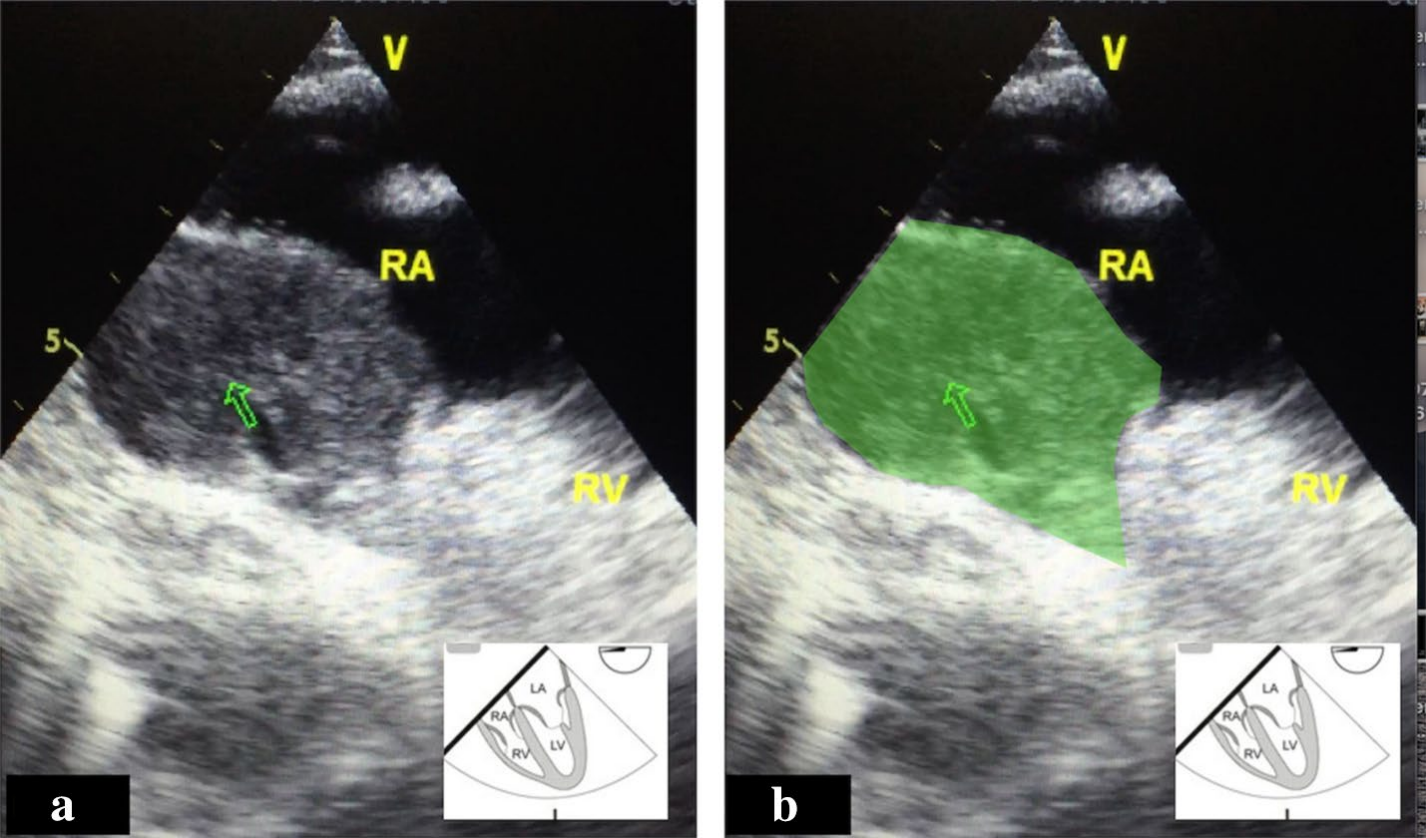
a, b Transesophageal echocardiogram (TEE) at mid-esophageal 4-chamber view with probe rotating to the right showed a compressed right atrium and a posterior pericardial clot measuring (green watermark). *RA* right atrium, *RV* right ventricle
